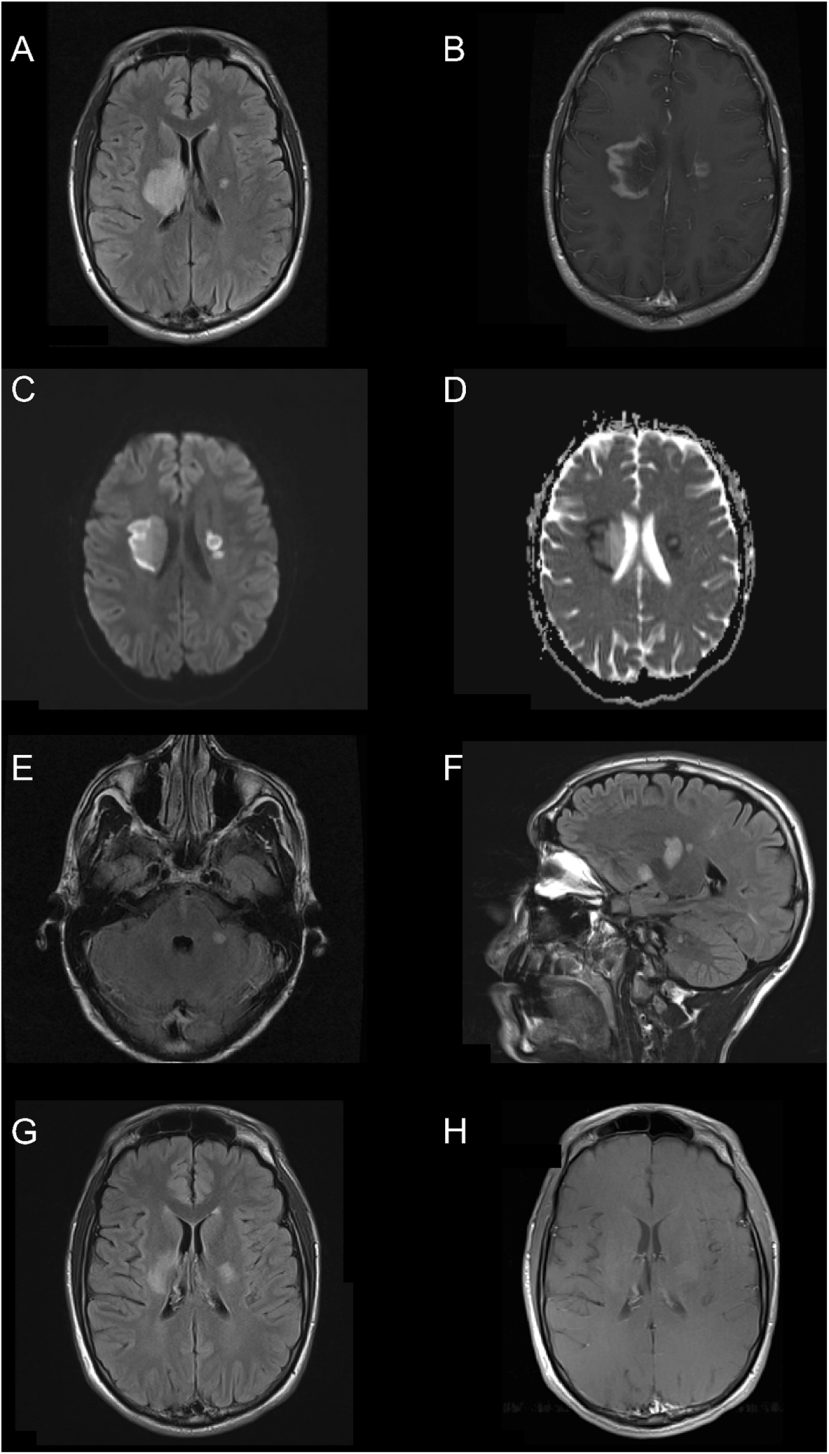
Case 1: MR images of brain lesions. a On admission a large area of increased FLAIR signal is present in the right lentiform nucleus, internal capsule, thalamus, corona radiata and centrum semiovale and a small signal focus is present in the left internal capsule/globus pallidus region. b A rim of gadolinium enhancement is present. c Restricted diffusion is shown by diffusion-weighted imaging in conjunction with the ADC map (d). e and f Five days later FLAIR images show additional lesions in the right medial pons, left cerebellar peduncle, putamen and corona radiata. Five months later, all lesions are smaller (g) and non-enhancing (h)
